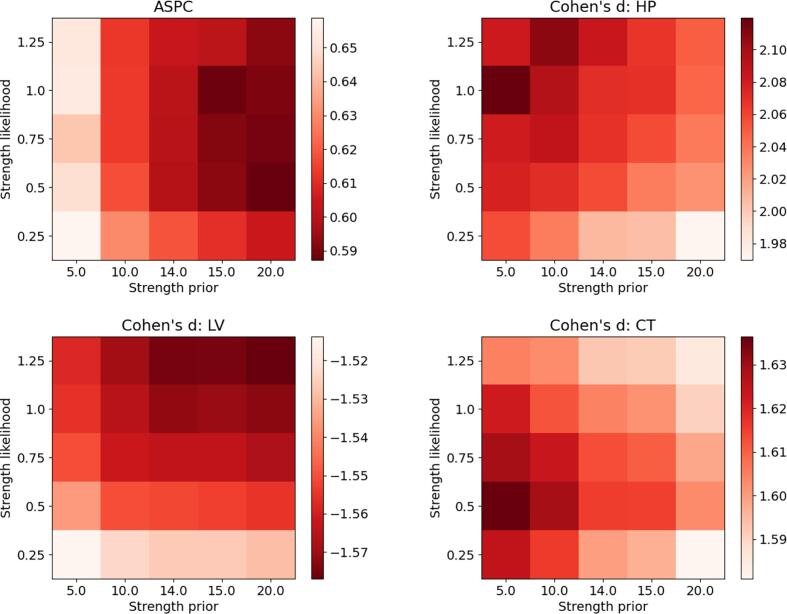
Hyperparameter tuning by grid search over the hyperparameters of the proposed method. Top left: ASPC values across subjects and structures for the 80 test–retest T1w scans of the 40 subjects of the MIRIAD-TR-HT dataset (CN=10, AD=30). Top right and bottom row: Cohen’s effect sizes computed from APCs estimates of the 80 subjects of the ADNI-HT dataset (CN=37, AD=53) for Hippocampus (HP), Lateral Ventricles (LV) and Cerebral Cortex (CT). APSC=Absolute Symmetrized Percent Change, CN=Cognitive Normal, AD=Alzheimer’s Disease.
